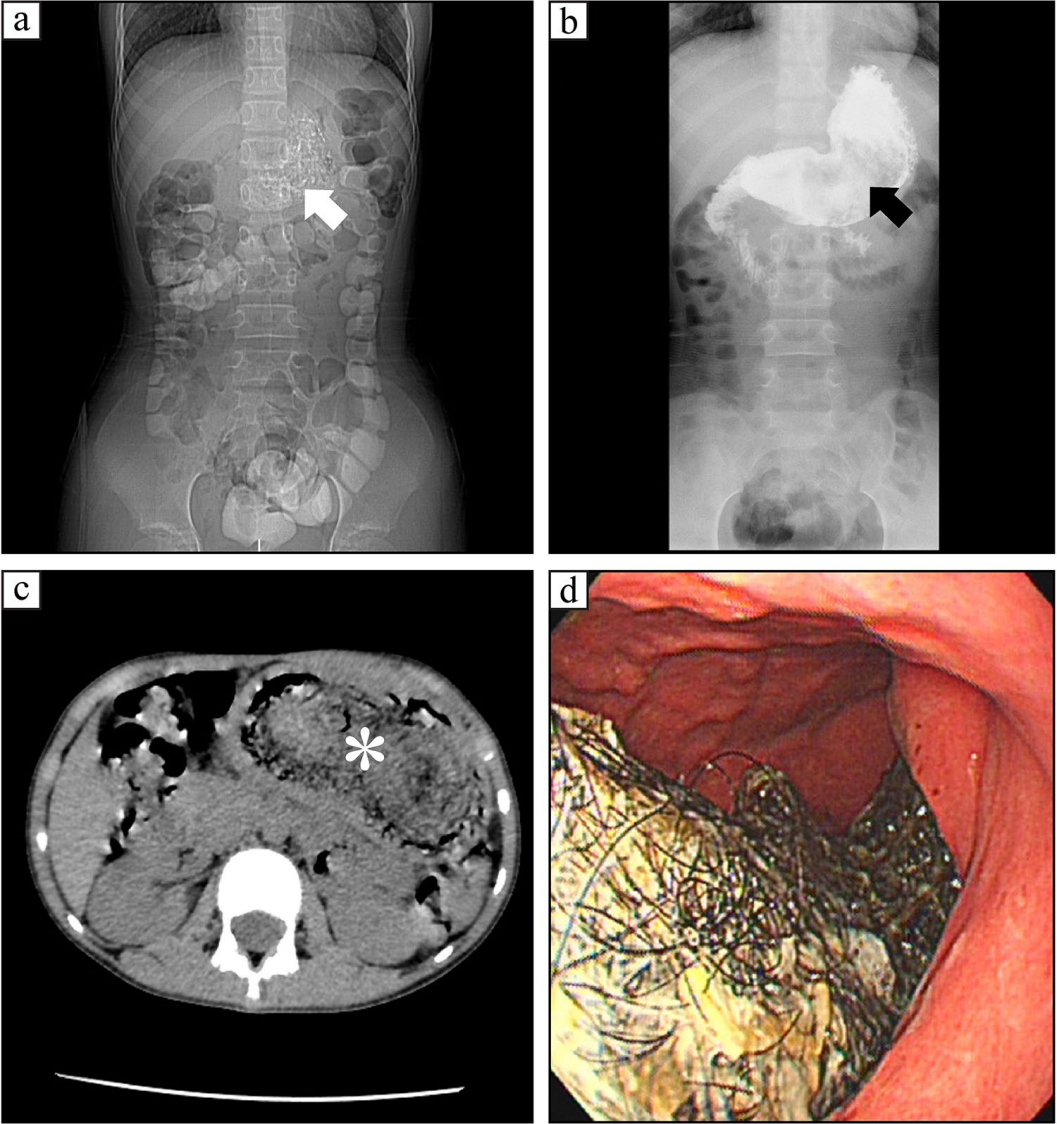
Gastric trichobezoars. a: Abdominal plain film showing a heterogeneous density mass in the left upper quadrant (white arrow head); b: GI demonstrating a gastric filling defect (black arrow head); c: CT scanning displaying a miscellany density mass in the stomach (asterisk); d: Gastroscopy revealing a hair mass in the stomach and duodenum. *GI* Upper gastrointestinal study, *CT* computed tomography
